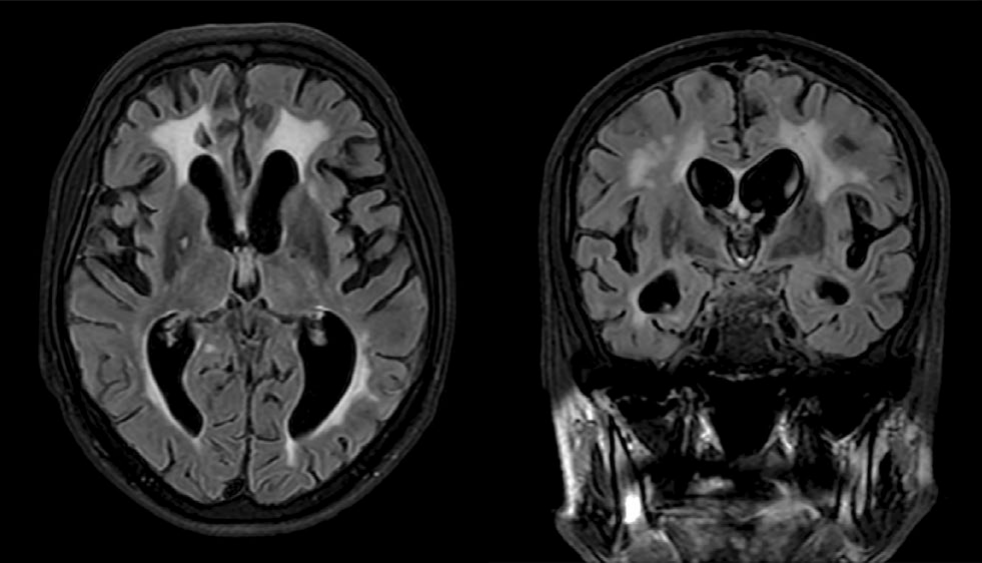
FIGURE 1 Brain MRI severe brain atrophy, mainly hippocampus and temporal lobes. Also hydrocephalus of chronic characteristics, with confluent T2 hyperintensity affecting the periventricular white matter, related to demyelination of probable chronic hypoxic origin
